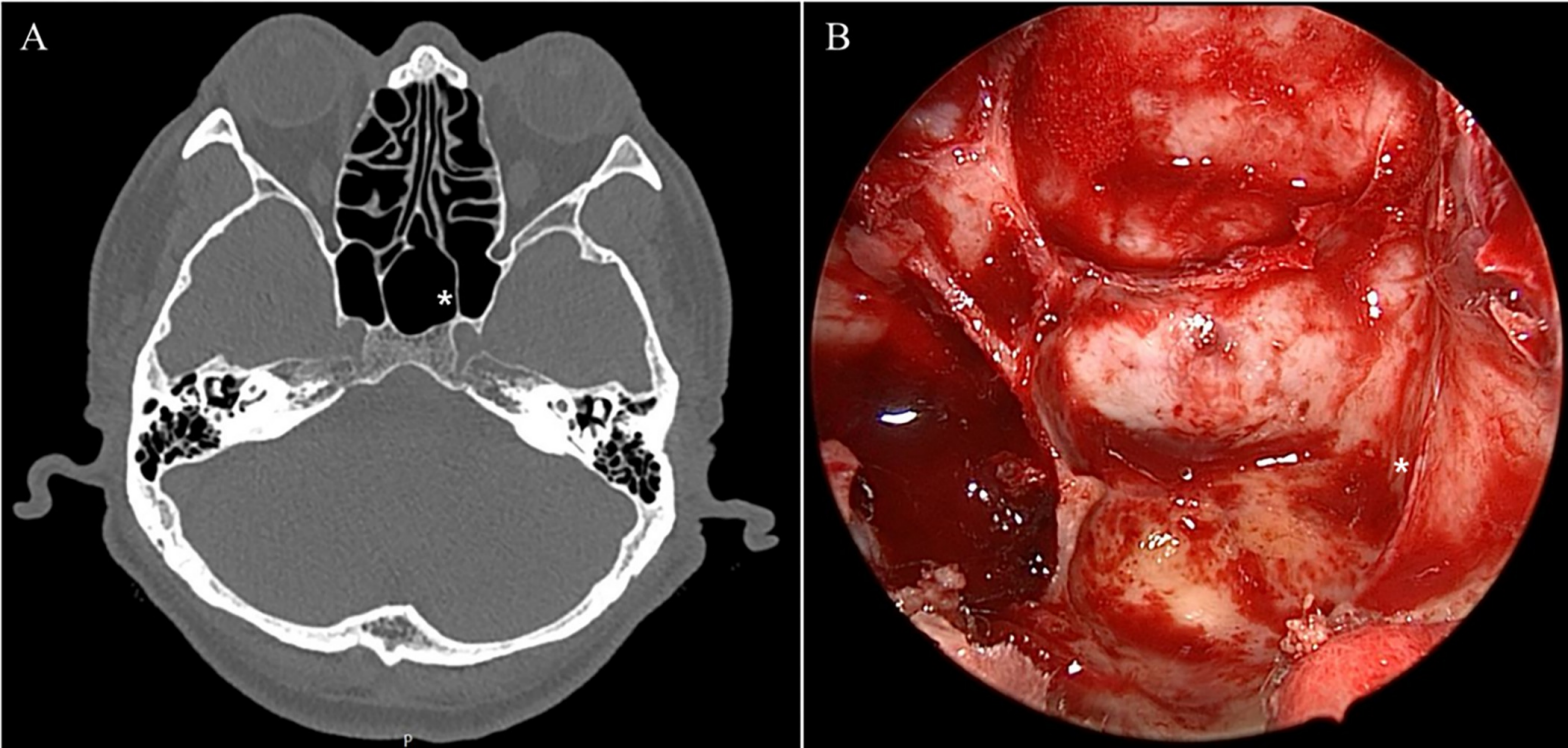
An intersphenoid sinus septum inserted into the paraclival internal carotid artery (asterisk) in a patient with presellar pneumatization, as revealed by paranasal sinus computed tomography (A) and during endoscopic endonasal transsphenoidal surgery (B).
